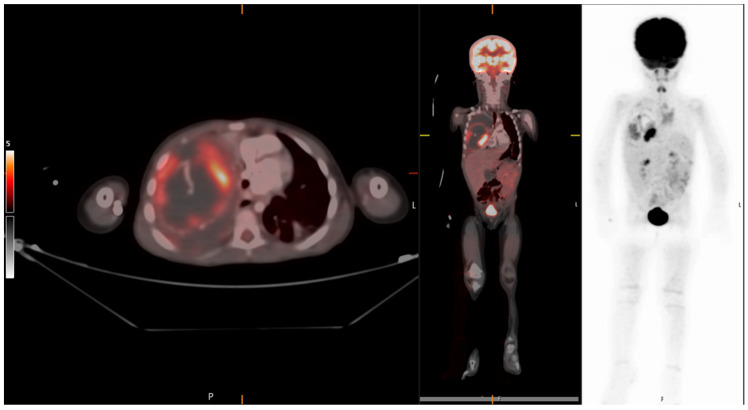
The same patient as in image 4. FDG PET-CT in the axial and coronal hybrid images and coronal PET image show peripheral uptake in the lung lesion. No metastases seen.
